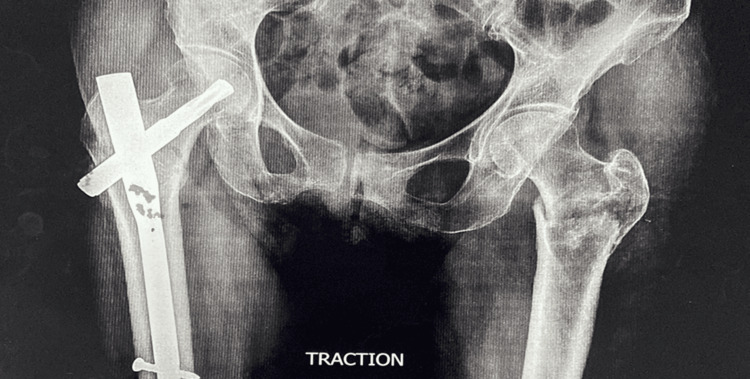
Plain radiograph of the pelvis with hips showing an operated case of a left intertrochanteric fracture with in situ PFNA2 and right-sided intertrochanteric fracture (Boyd and Griffin classification type 1) PFNA2: proximal femoral nail anti-rotation Asia
